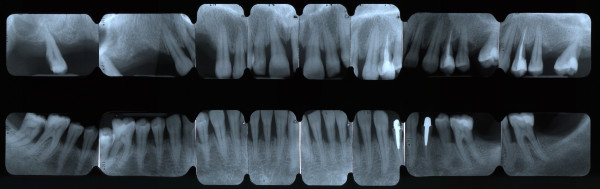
A full-mouth X-ray series. It is an important diagnostic support in periodontal patients (14/16 periapical X-rays) since it creates a full view of the patient's teeth and surrounding bone tissue.
